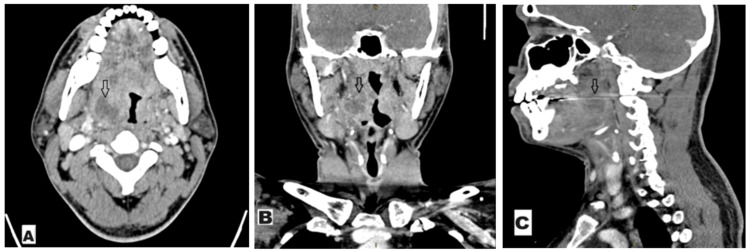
CECT of the neck demonstrating hypodense collection within the right palatine tonsil, measuring approximately 2.0 x 2.2 x 2.7 cm. A) A CECT of the neck axial cut with an arrow showing the right palatine tonsil collection; B) a CECT of the neck coronal cut with an arrow showing the right palatine tonsil collection; C) a CECT of the sagittal cut with an arrow showing the right palatine tonsil collection CECT: contrast-enhanced computed tomography
